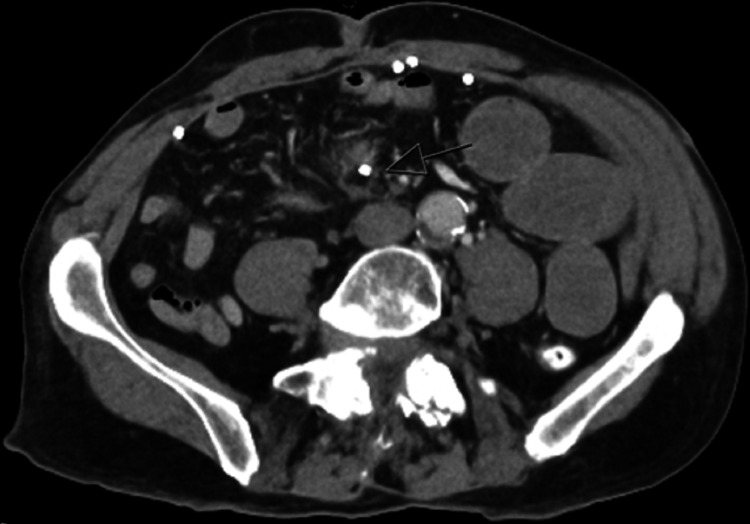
Axial CT image with an arrow showing the loose tack at the transitional point.
